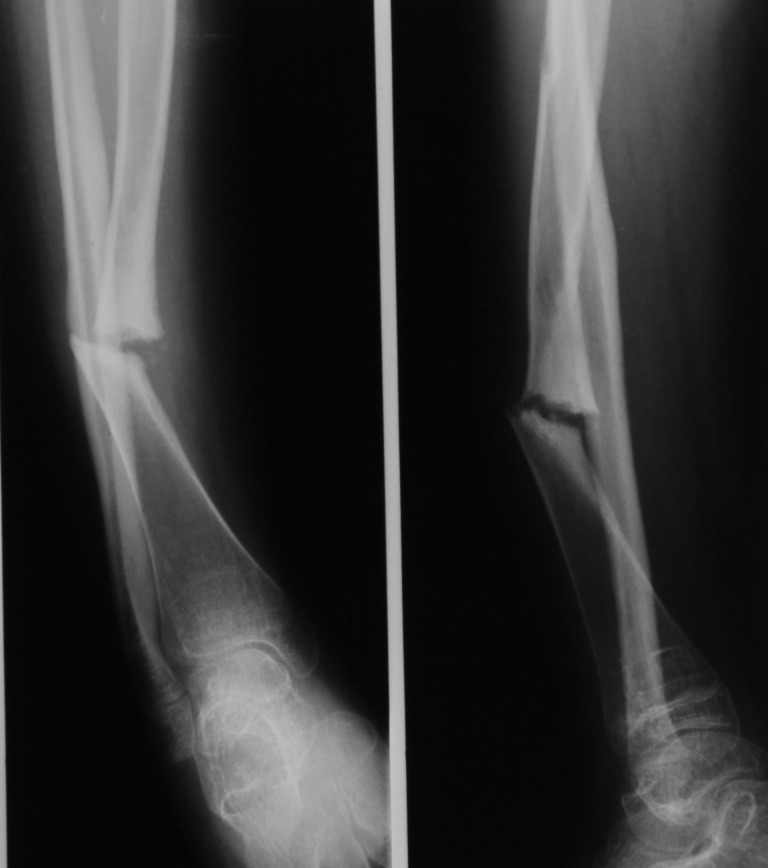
Preoperative X-ray image of case 2, twelve-year-old girl presenting a congenital pseudarthrosis of right tibia, Crawford IV.
